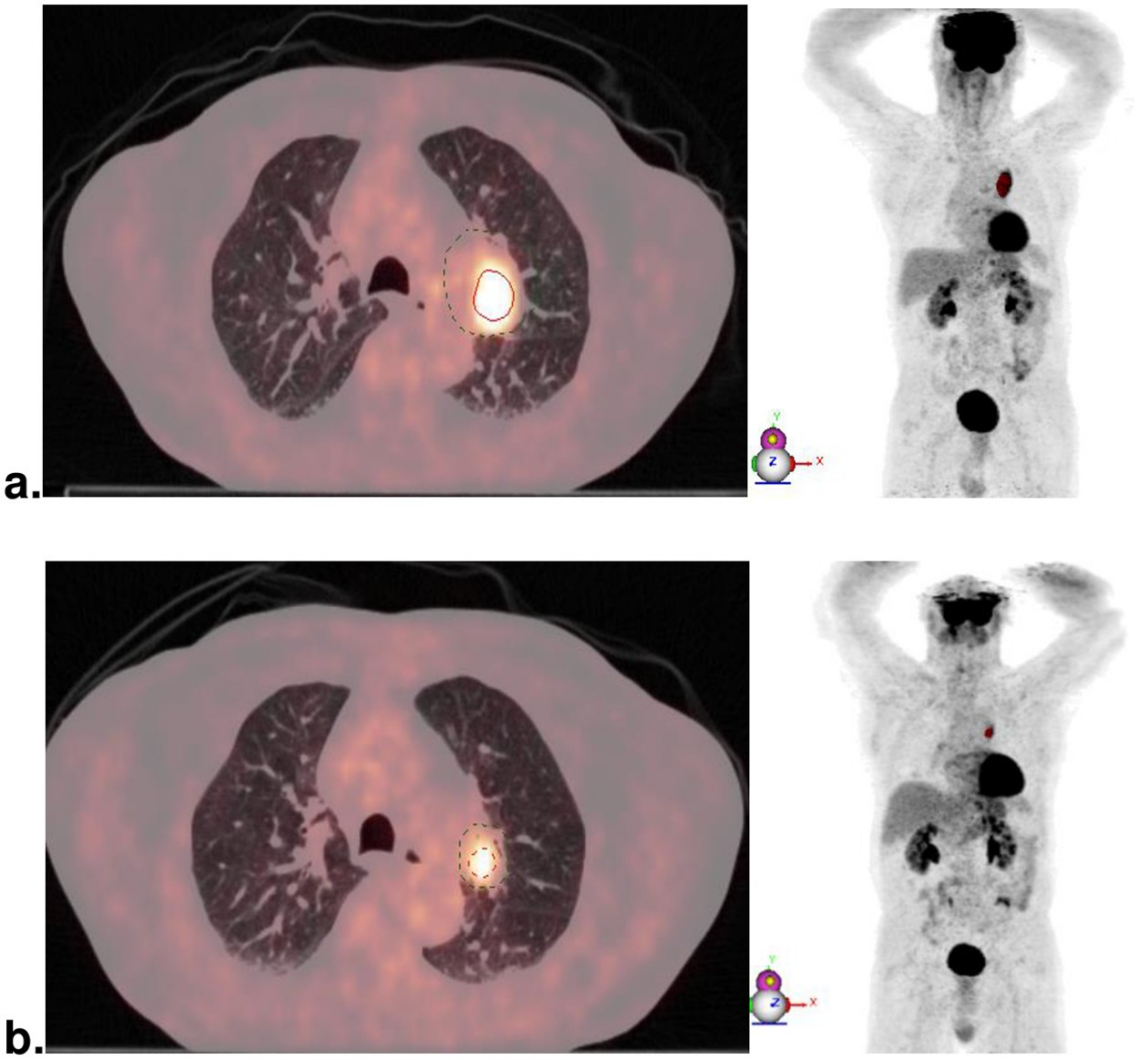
Tumoral response. 66-year-old patient with T_2_N_2_ (Stage IIIA) left upper lobe squamous cell carcinoma. At baseline (a), tumor SUVmax was 12 g/ml, MTV 13 cc and TLG 89 g. After 2 cycles of carboplatin-paclitaxel (b), SUVmax was 8.2 g/ml (ΔSUVmax = -33%), MTV 2.4 cc (ΔMTV = -82%) and TLG 11.3 g (ΔTLG = -87%). After 45 months, the patient was still alive and showed no sign of recurrence.
